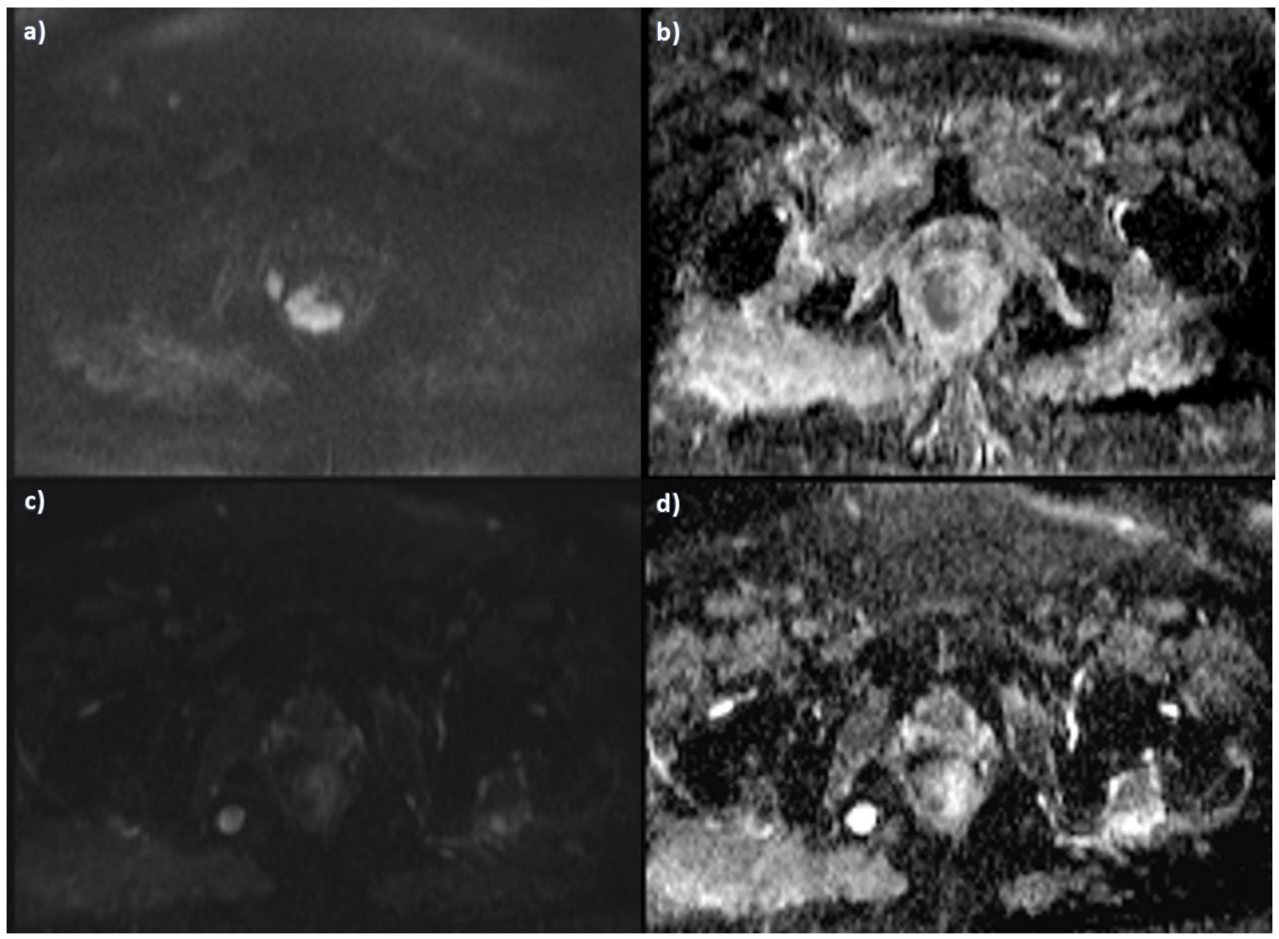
The same patient of Figure 3 Pre-treatment DWI images, in a. b800 s/mm2 the lesion shows restricted signal in b. ADC map with hypointense signal of the lesion. Post treatment assessment with a lower SI in b 800 s/mm2 c. and higher SI in ADC map d.
